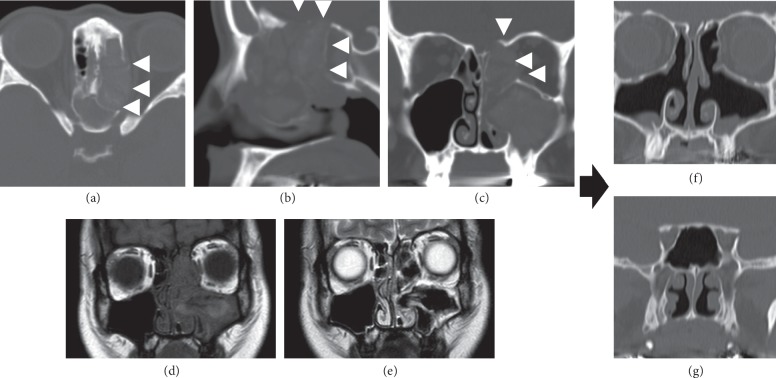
Case 2 MRI findings. (a) Computed tomography (CT) image of the paranasal cavity (axial section). (b) CT image of the paranasal cavity (sagittal section). (c) CT image of the paranasal cavity (coronal section). Soft-tissue contrasts are evident in the left maxillary sinus, the left frontal sinus, the left ethmoid sinus, and both sphenoid sinuses, along with bone thinning at the lamina papyracea and the base of the skull (white arrow). (d) T1-weighted MRI of the paranasal cavity (coronal section). (e) T2-weighted MRI of the paranasal cavity (coronal section). The paranasal sinus mucosa and nasal mucosa show isosignal intensity in the T1-weighted images, high signal intensity in the T2-weighted images, and the most of the allergic mucin show none to low signal intensity. (f, g) CT images of the paranasal cavity after the surgery (coronal section). There is no apparent evidence indicating disease recurrence.
